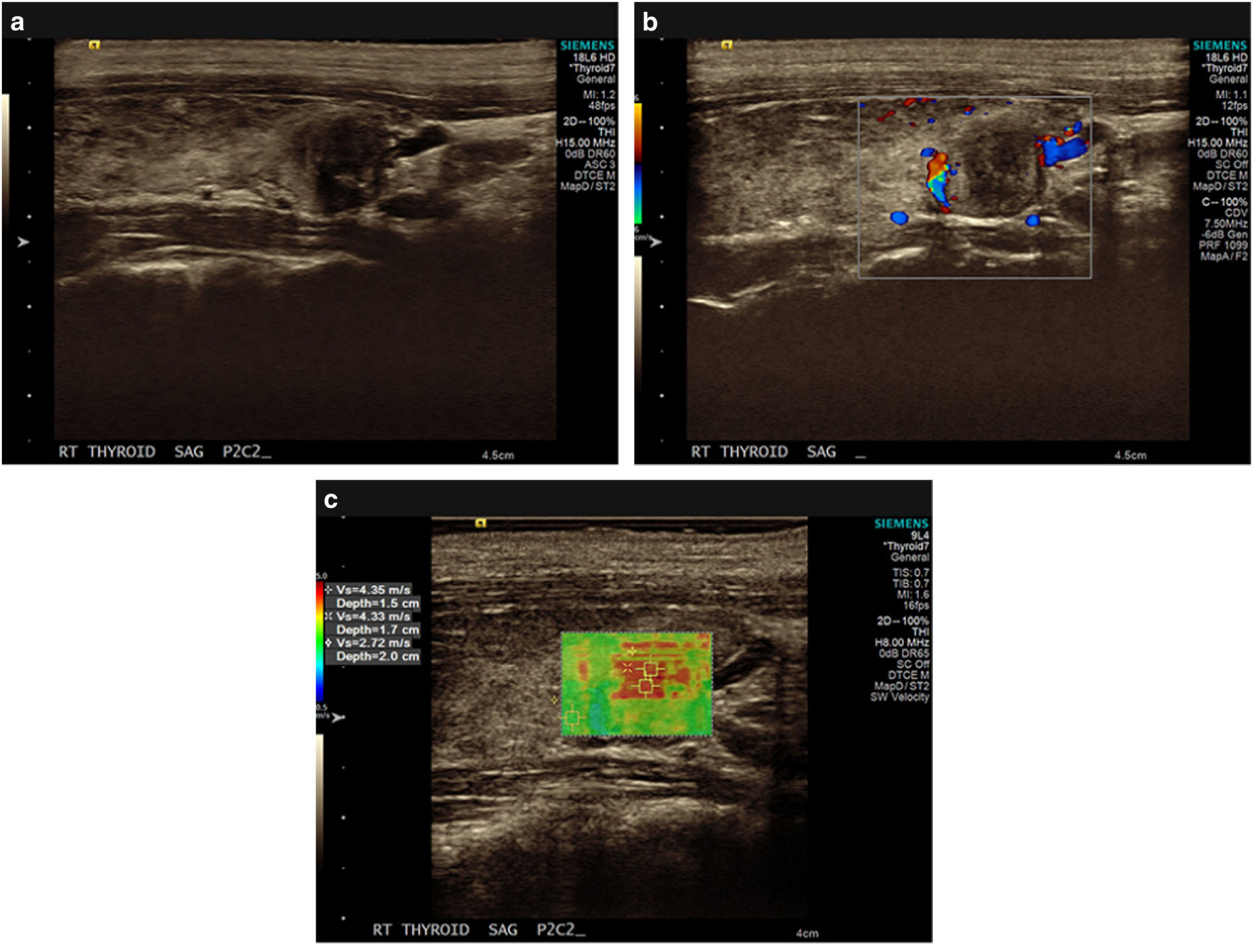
a Shows a B-mode image of a 12.1 × 11.4 × 9.1 mm thyroid nodule in the right lobe. b Shows Color Doppler vascularity and c shows SWV measurements. FNAB pathology showed Atypia of Undetermined Significance (BC III) and GEC-suspicious results. Surgical pathology confirmed a 10 × 8 × 5 mm FVPTC
